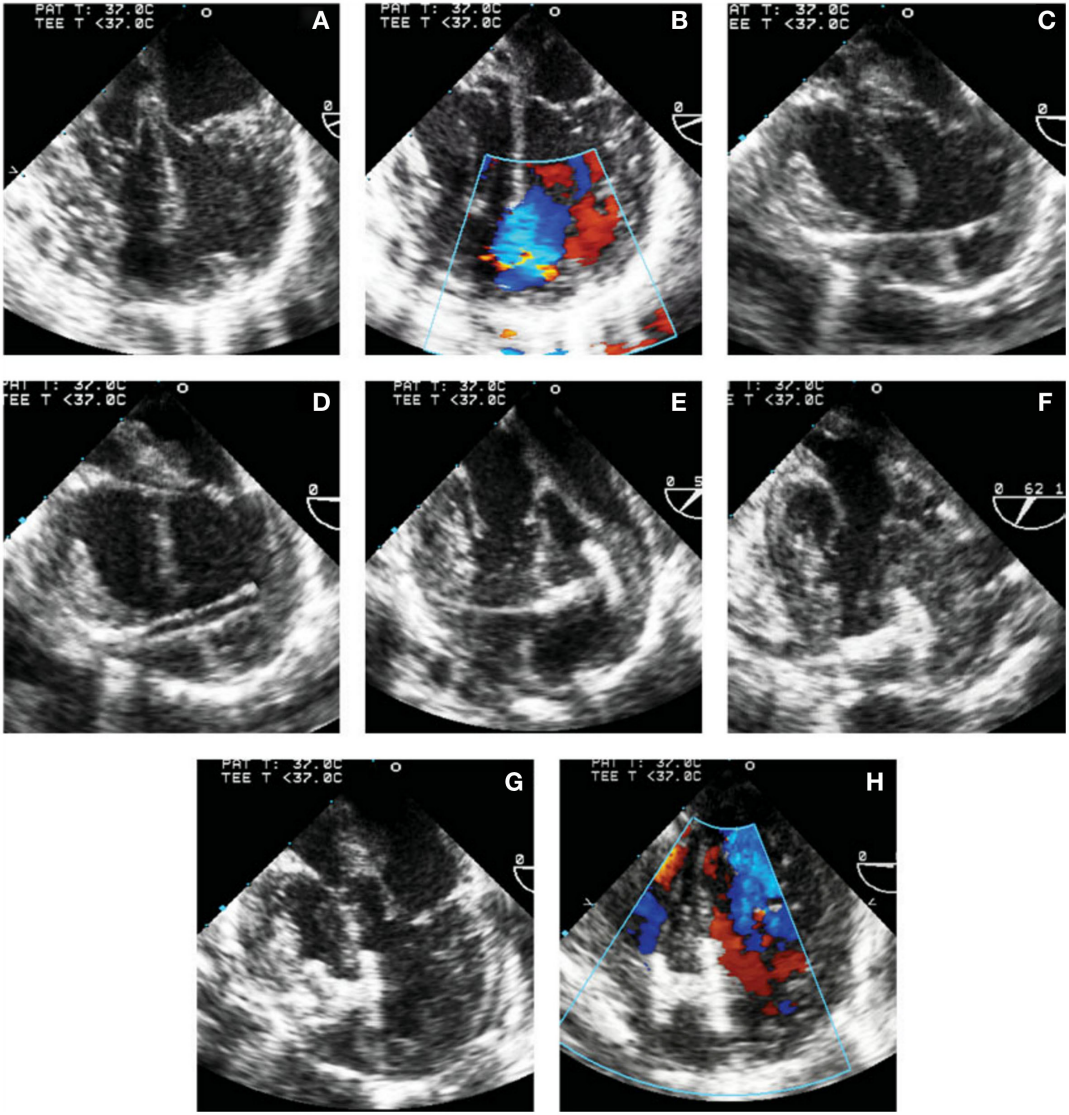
Transesophageal echocardiographic images of steps involved in perventricular ventricular septal defect (VSD) closure. (A,B) 4-chamber view without (A) and with (B) color Doppler showing the mid muscular VSD. (C) Wire through the right ventricular free wall and across the defect. (D) Delivery sheath in the left ventricle (LV) cavity. (E) Left disk deployed in the LV. (F) Left disk aligned with the ventricular septum. (G) Device waist and part of the right disk have been deployed. (H) Complete deployment of the right disk with optimal device position. *Reprinted with permission from publisher: John Wiley and Sons* (29).
